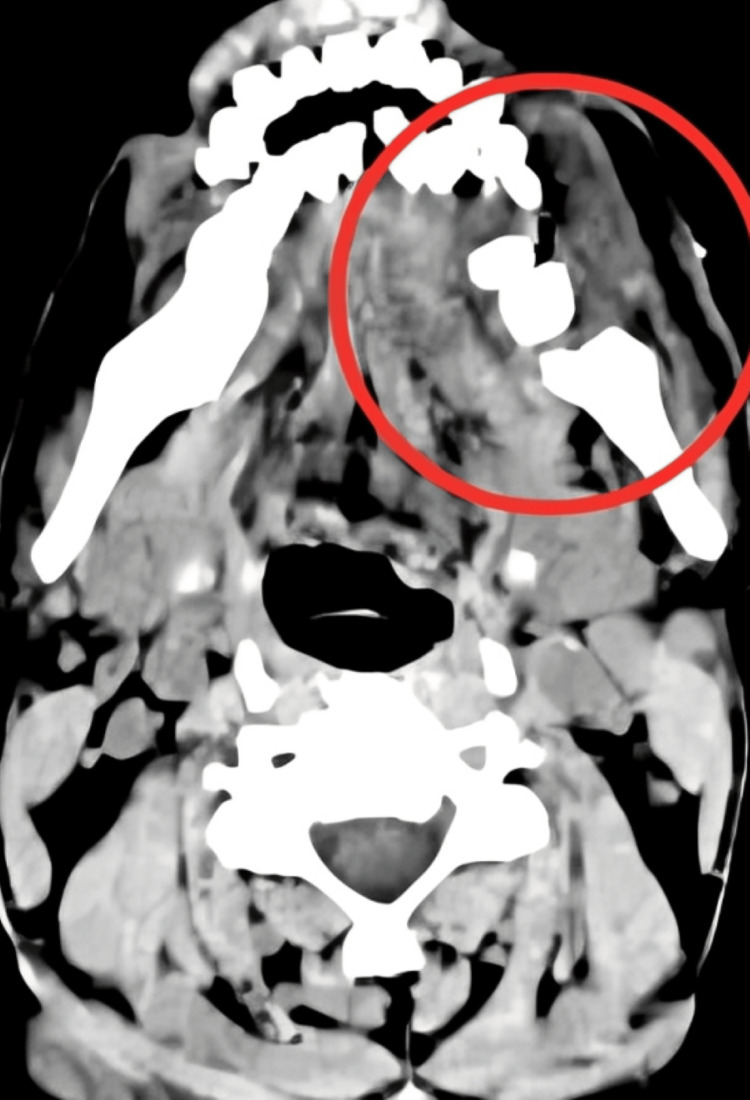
CECT after segmental mandibulectomy CECT: contrast-enhanced computed tomography
